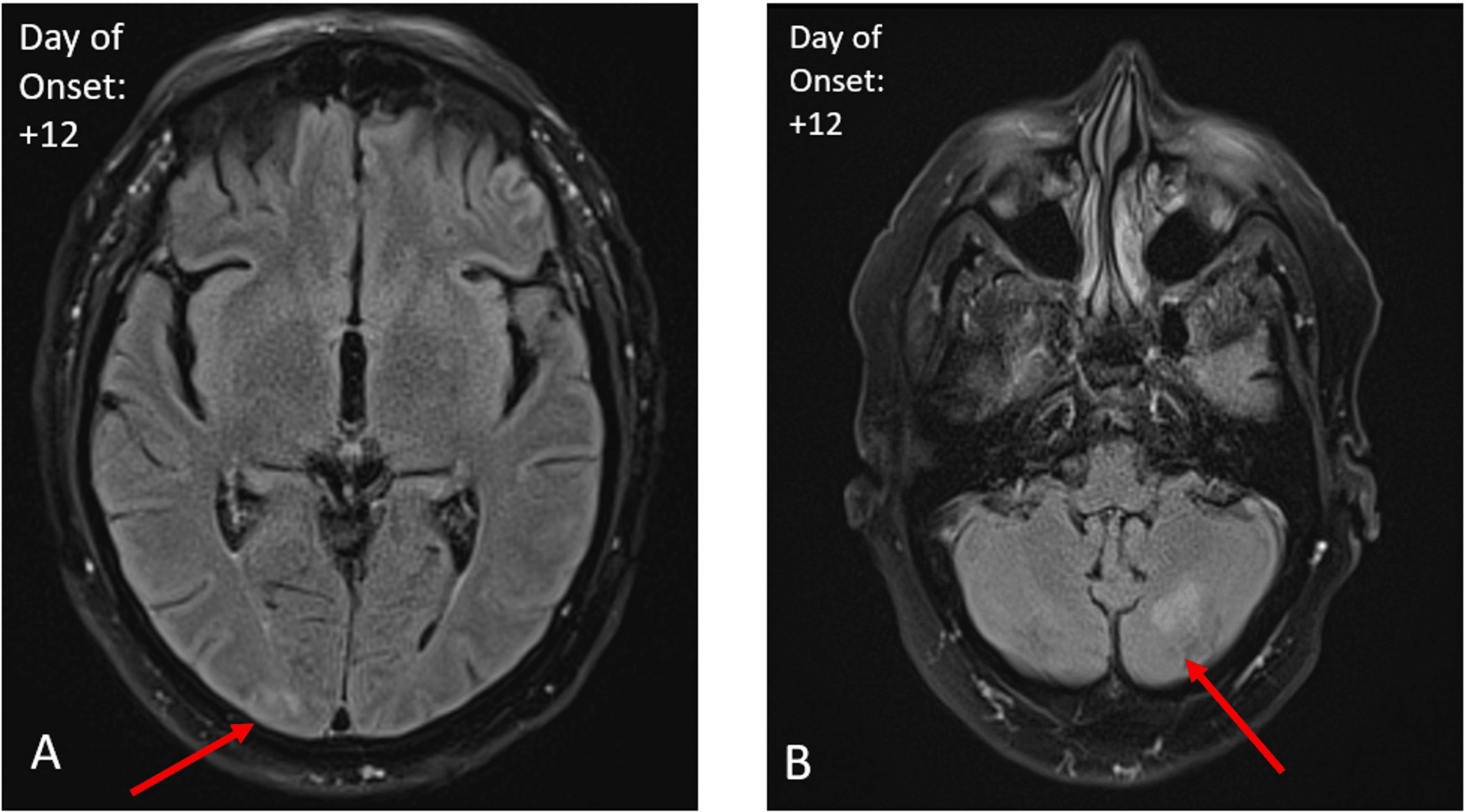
Follow-up axial fat-suppressed T2 FLAIR MRI. Day 12 after the onset of symptoms demonstrating mild sulcal FLAIR hyperintensity in the right occipital lobe (A) and patchy FLAIR hyperintensity in the left cerebellar hemisphere (red arrows) (B). FLAIR: fluid-attenuated inversion recovery.
